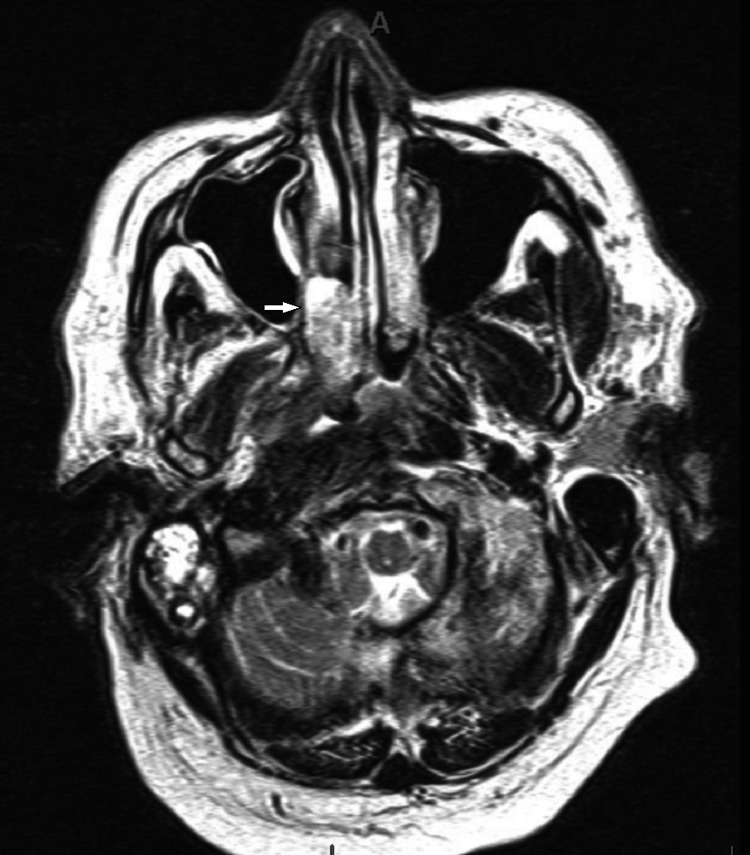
MRI of nasopharyngeal carcinoma Head magnetic resonance imaging of the neoplasm (arrow) shows an ill-defined infiltrating mass in the posterior right aspect of the nasopharynx extending into the skull base.
